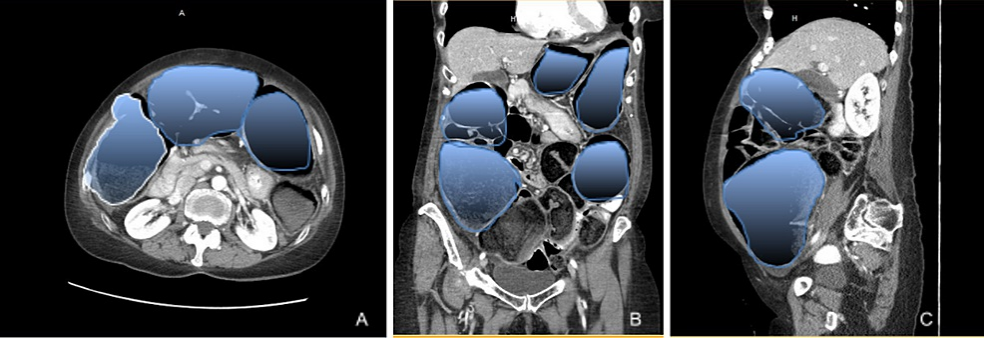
Non-contrast computed tomography axial view (A), coronal view (B), and sagittal view (C) of the abdomen showing dilated loops of large bowel within the abdominal cavity (area highlighted).
